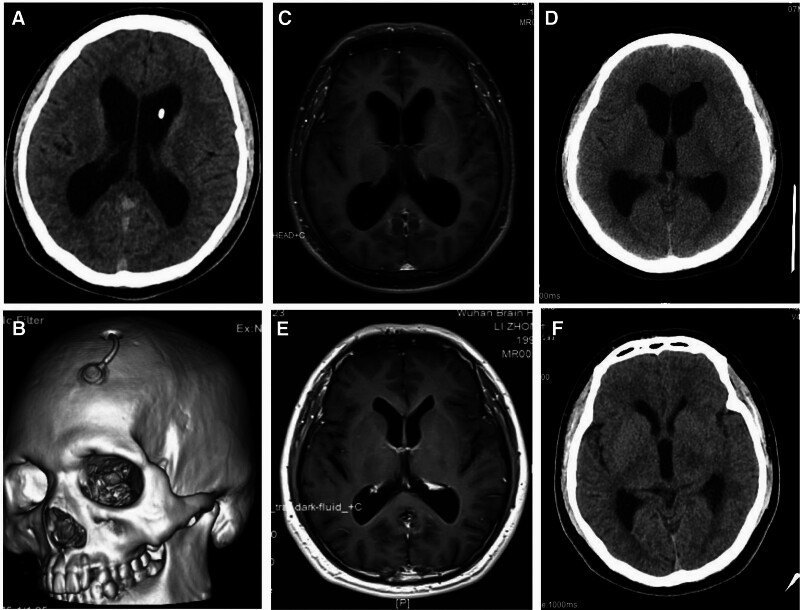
Postoperative CT scan. Head CT scan after surgery showed that (A and B) the drainage tube and Ommaya reservoir were well positioned. Head CT scan for recovery status at 1.5 (C), 5 (D), 11 (E), and 14 mo (F) postoperatively. CT = computed tomography.
